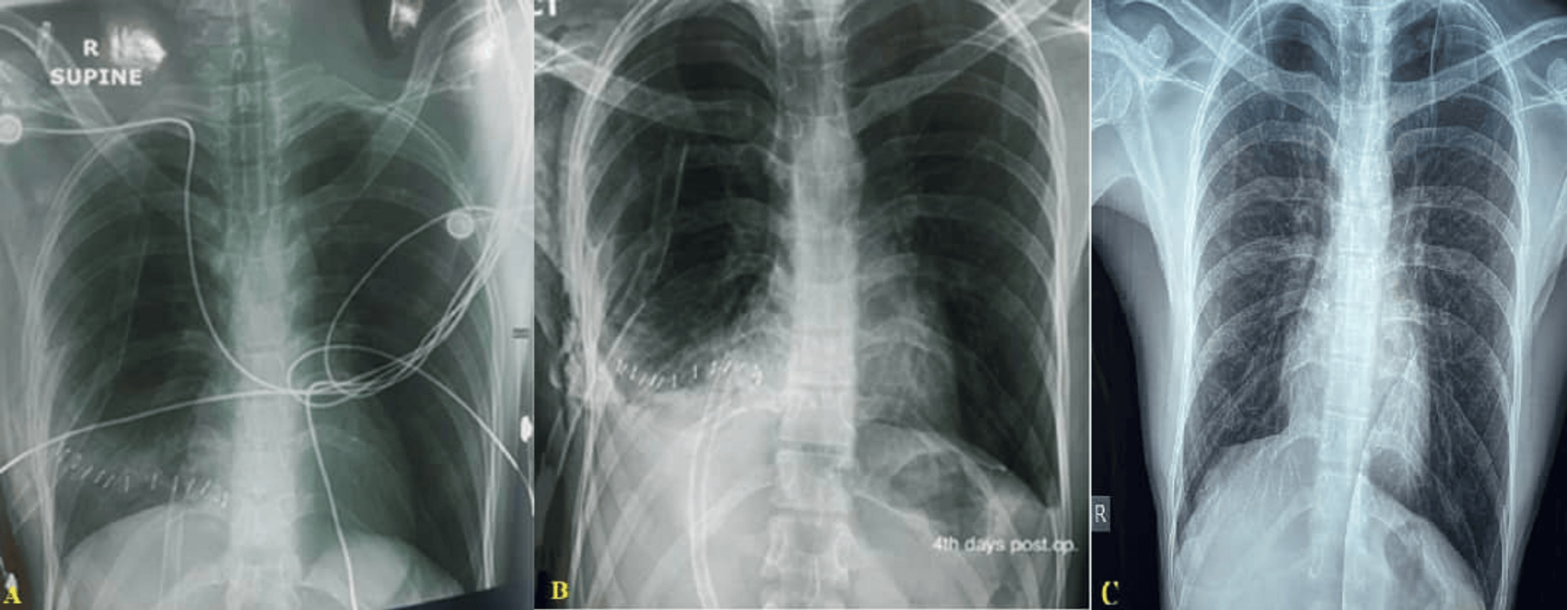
Postoperative radiologic X-ray showed no abnormalities (A) on the first postoperative day, (B) the fourth postoperative day, and (C) at the three-month follow-up
